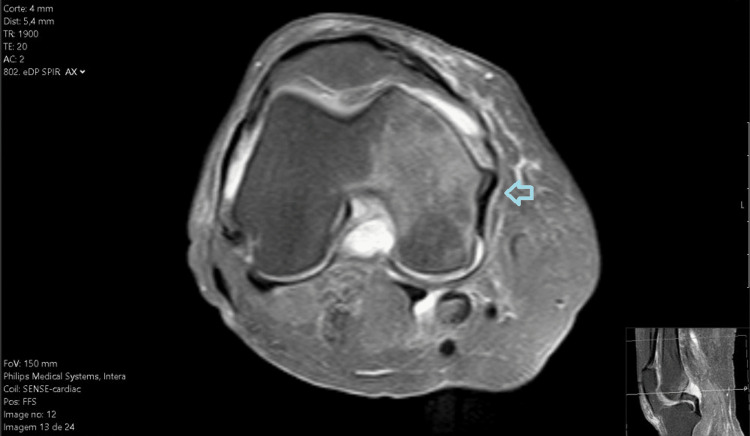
MRI of the right knee in the axial plane.
